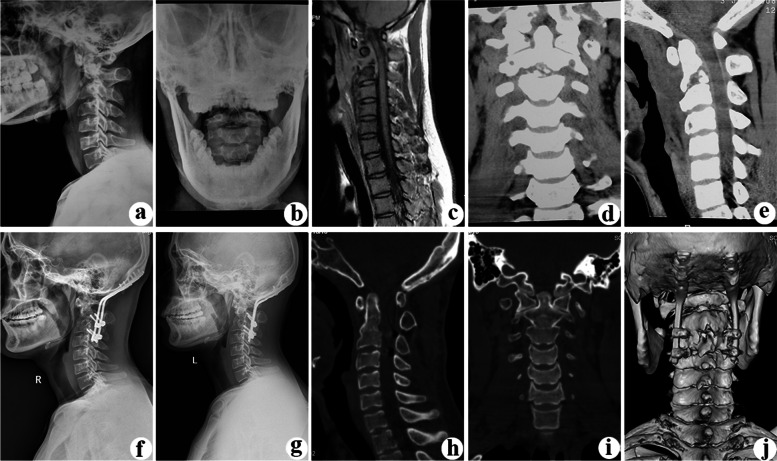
Endoscopy-assisted anterior cervical debridement combined with posterior fixation and fusion were performed for an 18-year-old man with upper cervical spine TB. Preoperative lateral (a) and open mouth (b) radiographs, MRI (c) and CT (d, e) show C2/3 vertebral destruction, spinal cord compression, and abscess formation. Postoperative X-ray examination (f) shows satisfactory posterior occipital-cervical fixation and a well-corrected cervical vertebral sequence. At the final follow-up at 72 months postoperatively, a radiograph (g) shows well-placed fixation, and CT (h–j) shows solid bony union
